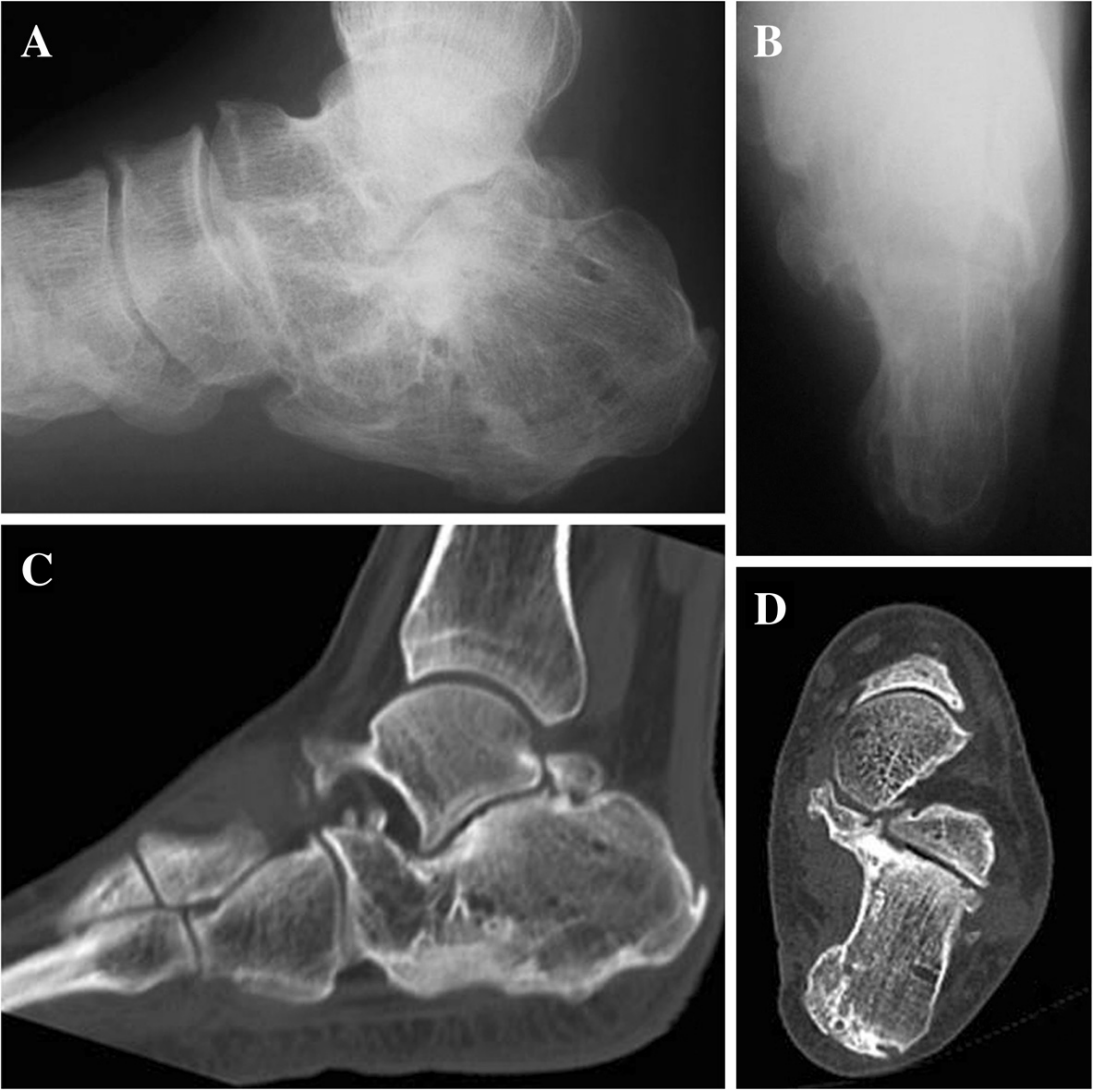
The radiographs (A, anteroposterior view; B, lateral view) and CT images (C, sagittal view; D, axial view) taken at the latest follow up demonstrate the congruent subtalar joint and degenerative changes.
